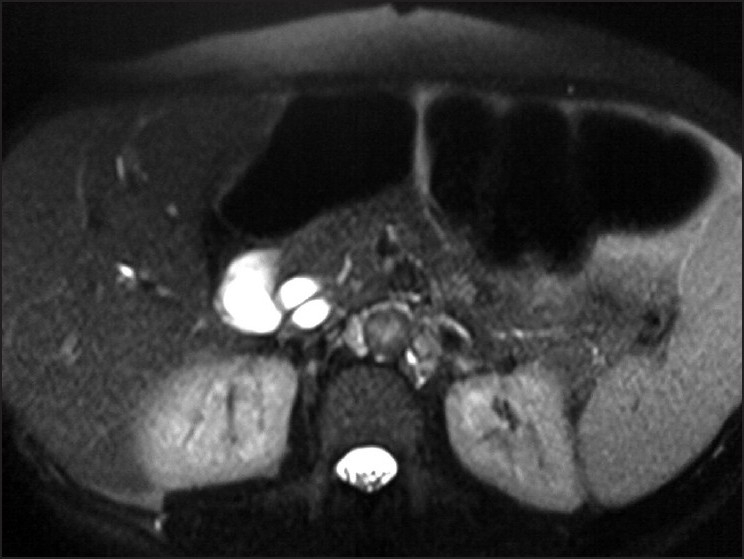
Axial heavily T2-weighted MR (MRCP) image showing closely related, parallel running common hepatic (CHD) duct (anterior) and cystic duct (posterior) in the region of pancreatic head
